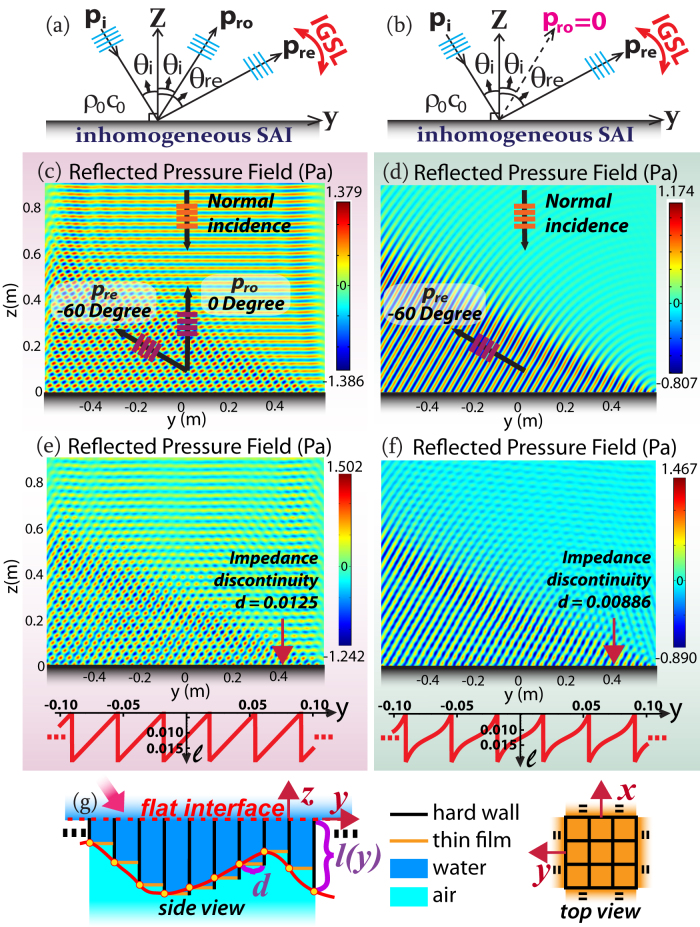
(a) For a flat interface with an inhomogeneous SAI, the angle of *p_ro_*, i.e., *θ_ro_*, is not influenced, while *p_re_* occurs simultaneously and *θ_re_* is controlled by IGSL. (b) If SAI is properly controlled, *p_ro_* is null. (c) Ultrasound with unit amplitude and *ω* = 300 *Krad*/*s* impinges upon SAI surfaces in water. The SAI along the flat surface generates both *p_ro_* and *p_re_* when an arbitrary *A* is chosen in Eq.(1). (d) A particular SAI is chosen according to Eq.(7).
